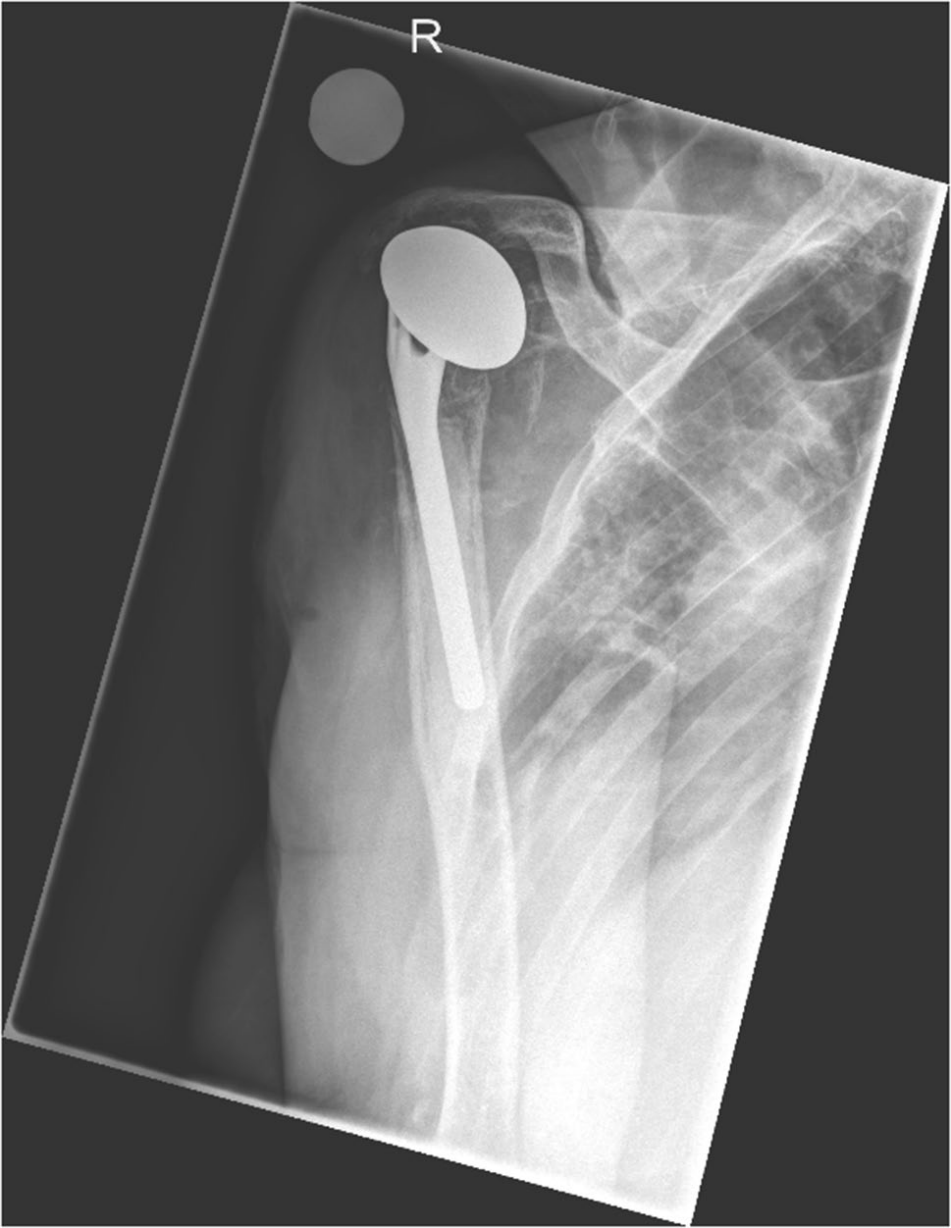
Radiographic examination of the right shoulder joint (a.p. view) prior revision surgery due to periprosthetic infection
